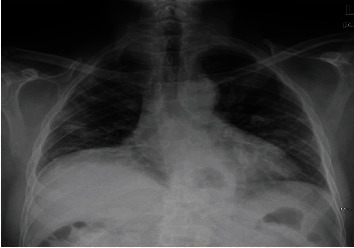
AP portable radiograph showing low lung volumes and patchy linear opacities in the periphery of both lungs.
